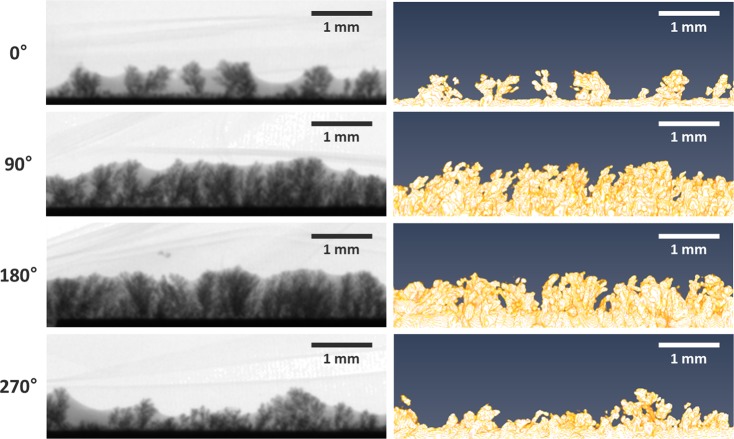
Magnified version of locally agglomerated electrodeposit at the surface of electrode in Fig. 5b. Radiographic images (left) and corresponding reconstructed CT images (right) are visually comparable even at different angular positions: 0°, 90°, 180°, and 270°.
